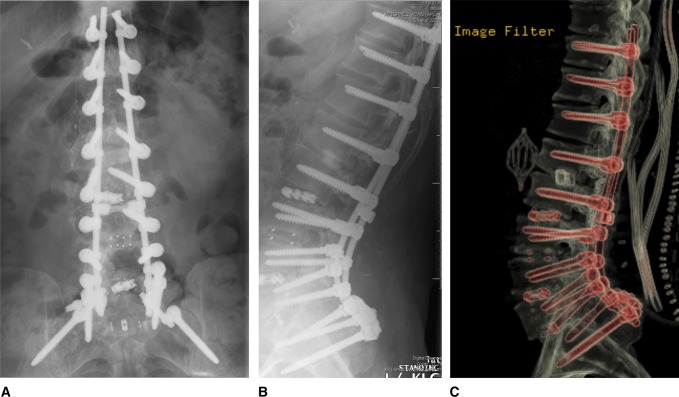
A and B, AP/lateral lumbar XR. C, CT implant series.
